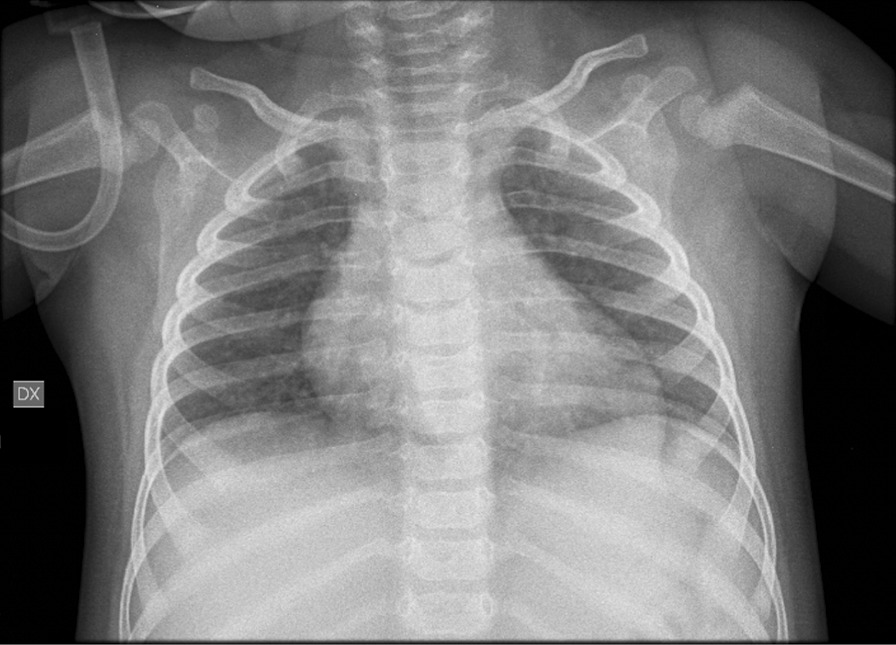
Patient’s chest X-ray A-P projection. Widespread mild accentuation of the broncho-vascular texture with blurred aspect
